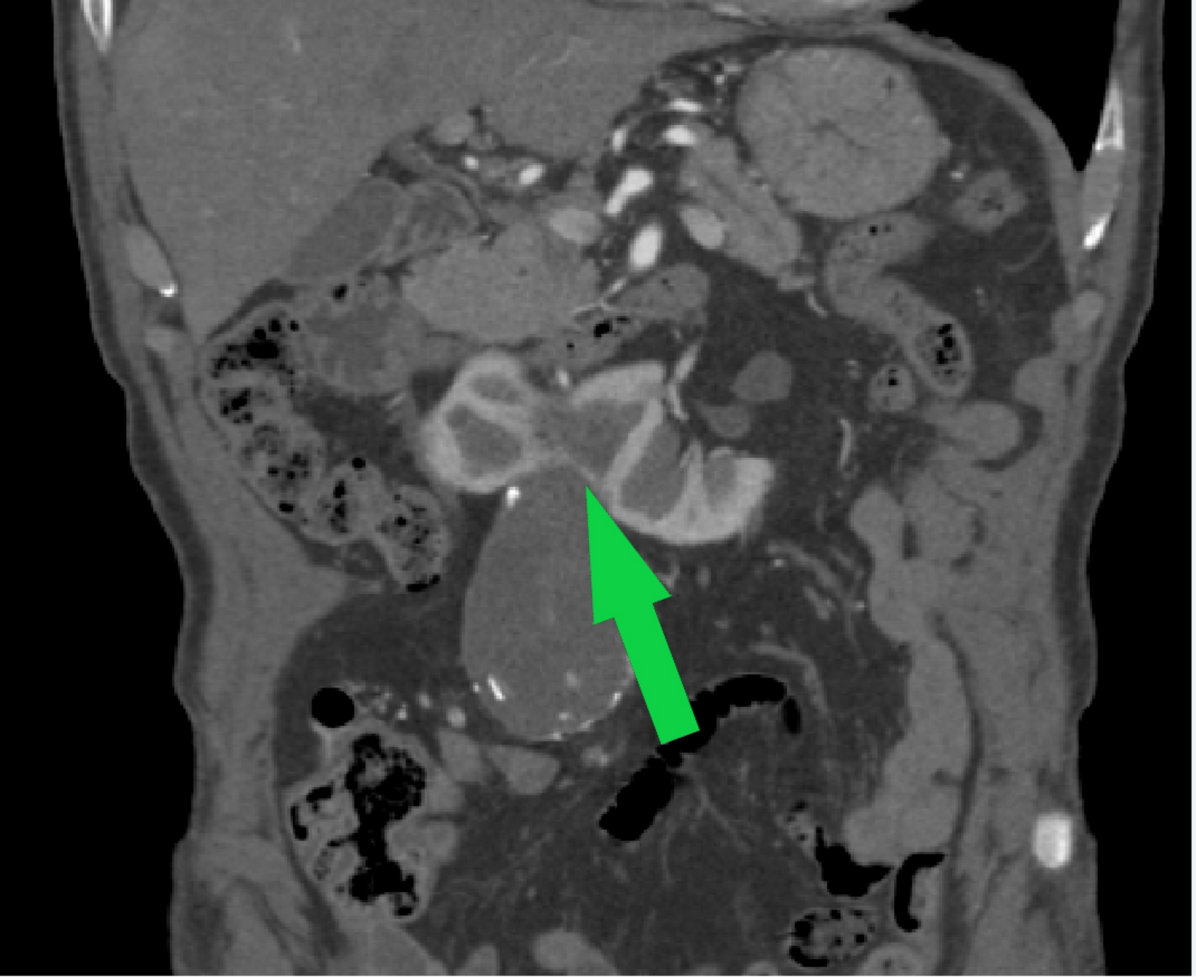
Preoperative abdominal CT angiography, coronal cross-section, demonstrating HSK (green arrow) HSK, horseshoe kidney
